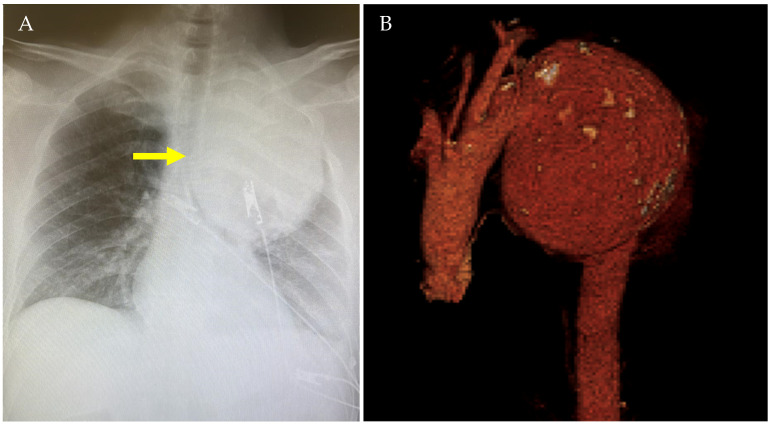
Chest radiography (A) and three-dimensional volume-rendered reconstruction of the pre-operative CTA (B) showing a 11.5 cm sacciform aneurysm of the distal aortic arch (yellow arrow).
